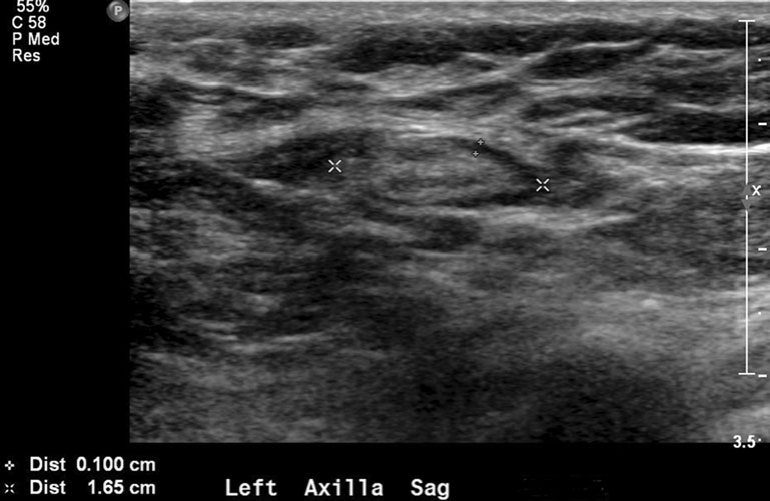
Normal lymph node: On ultrasound, lymph nodes typically are smooth, gently lobulated ovals with a hypoechoic cortex measuring less than 3 mm in thickness with a central echogenic hilum
